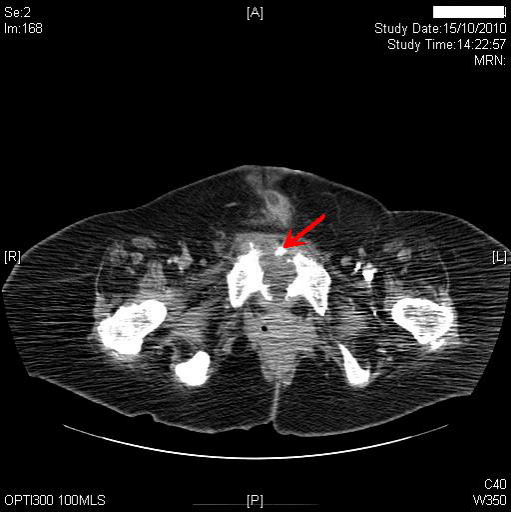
Computed tomography of abdomen, which was performed four months later, revealed erosion of symphysis pubis with a few bony fragments in the joint space (red arrow).
